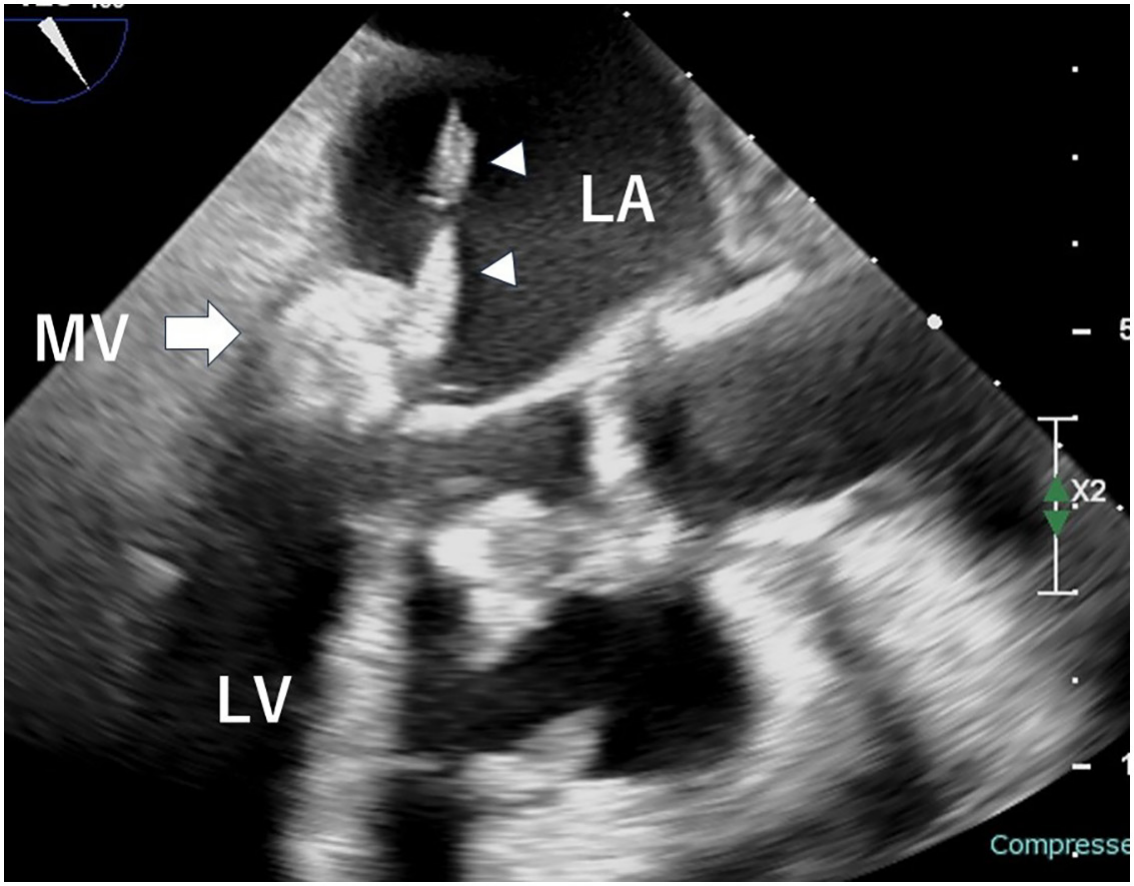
Transesophageal echocardiogram. A 3-cm long vegetation (arrowhead) was attached to the tumor-like posterior leaflet (arrow). LA, left atrium; LV, left ventricle; MV, mitral valve
